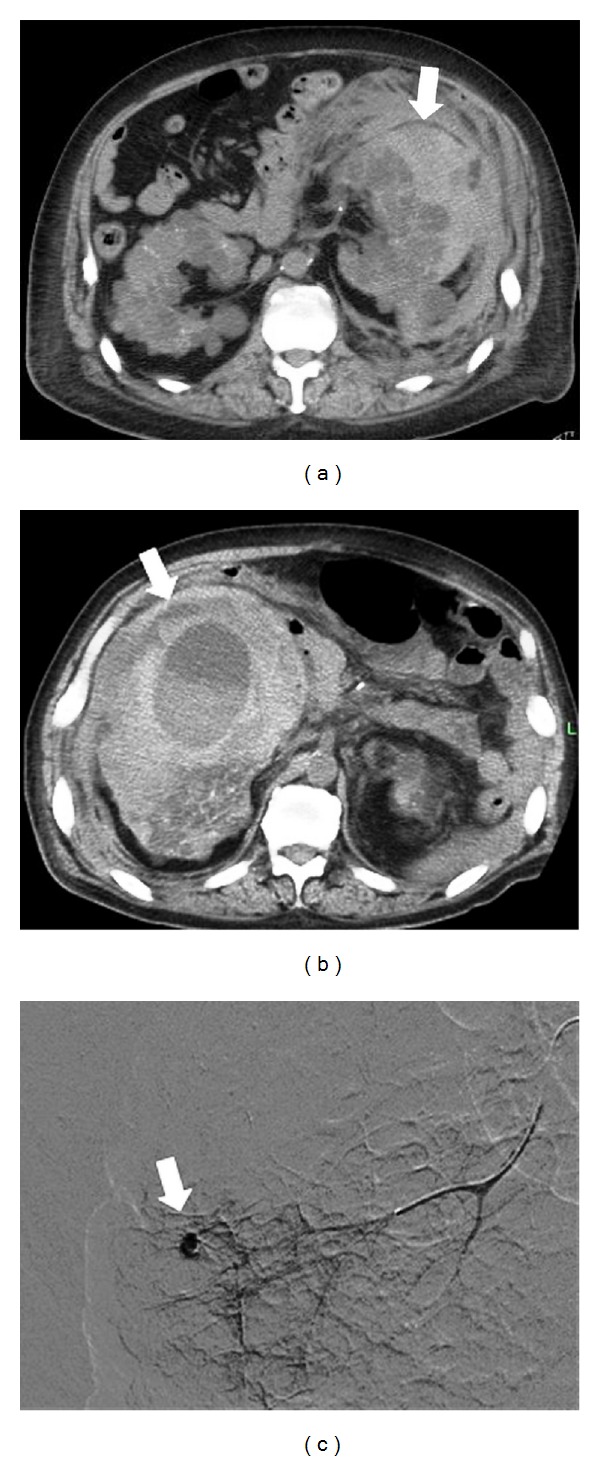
CT scan of the abdomen. (a) A large number of bilateral renal cysts were observed. A huge left perirenal hematoma (arrow) and (b) there was a large cyst including fluid with density of blood in right kidney, and a huge right perirenal hematoma (arrow). Renal arteriography during the right perirenal hemorrhage. (c) Extravasation from an aneurysm, being present at the periphery of right renal artery, was demonstrated (arrow).
